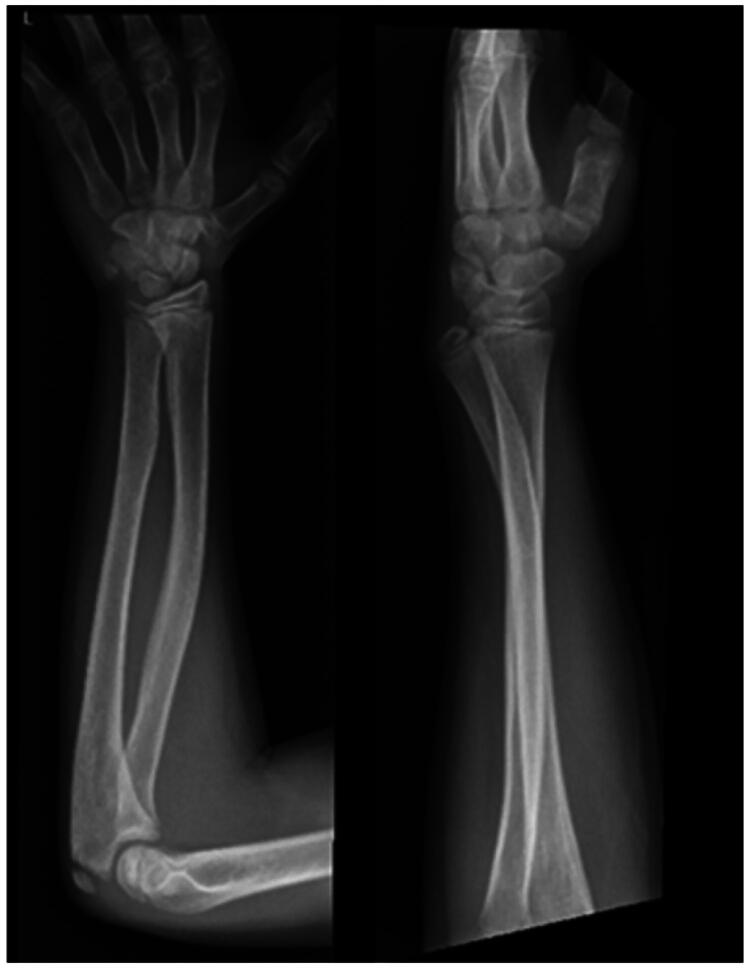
X-ray 8 years after injury showing marked angulation of both forearm bones and dorsal dislocation of the ulna in the DRUJ.
